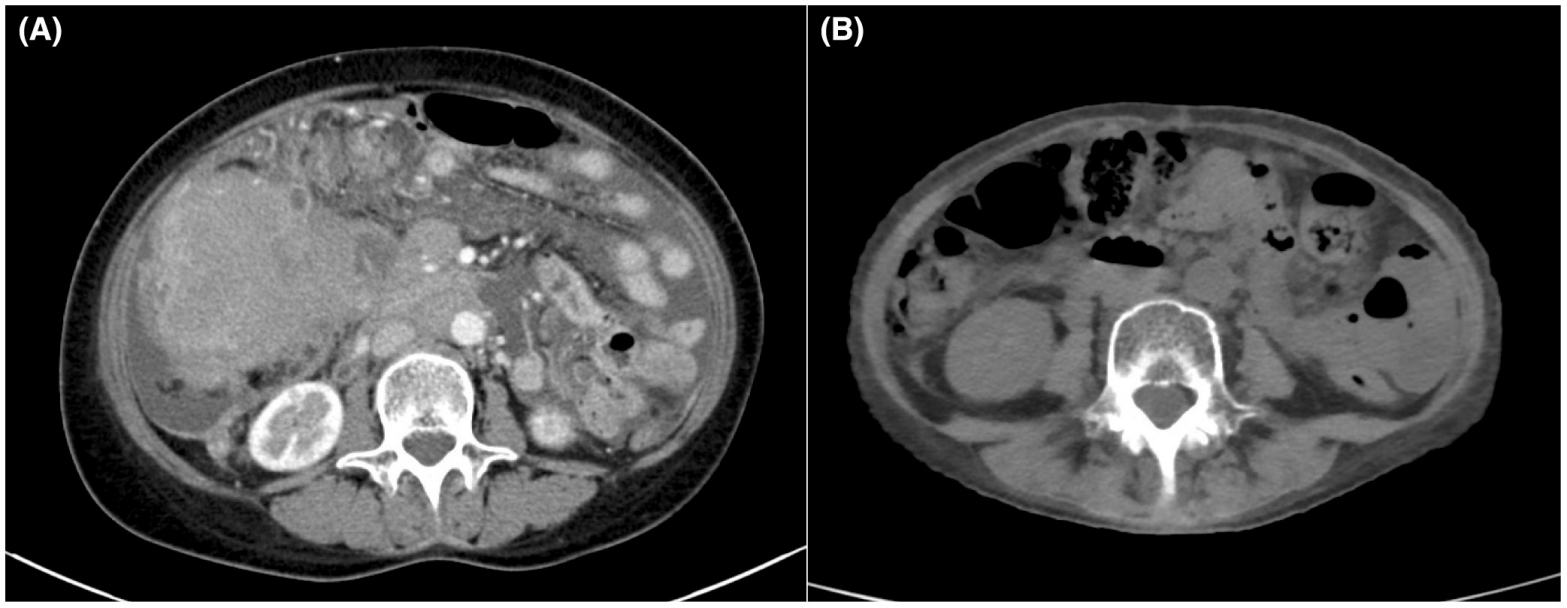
CT images of the abdomen.(A) Arterial phase CT before starting chemotherapy shows a tumor in the ileum and ascending colon. (B) Plane CT performed 7 months after the start of chemotherapy reveals regression of the tumor. CT: computed tomography.
